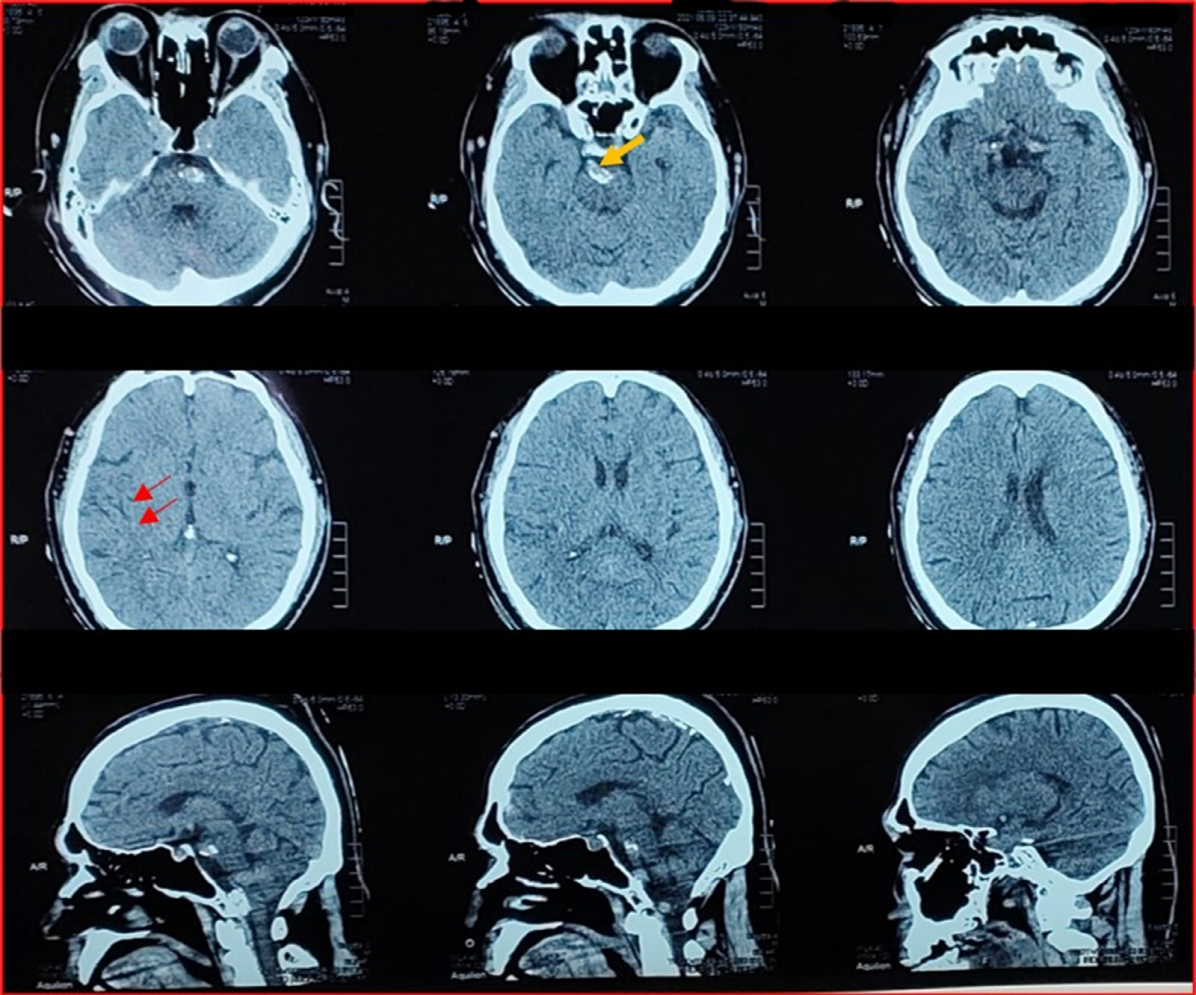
CT scan showed a blurred insular ribbon on the right side (red arrow). The ventral calcification of the pons is shown (orange arrow). (Color version of figure are available online)
